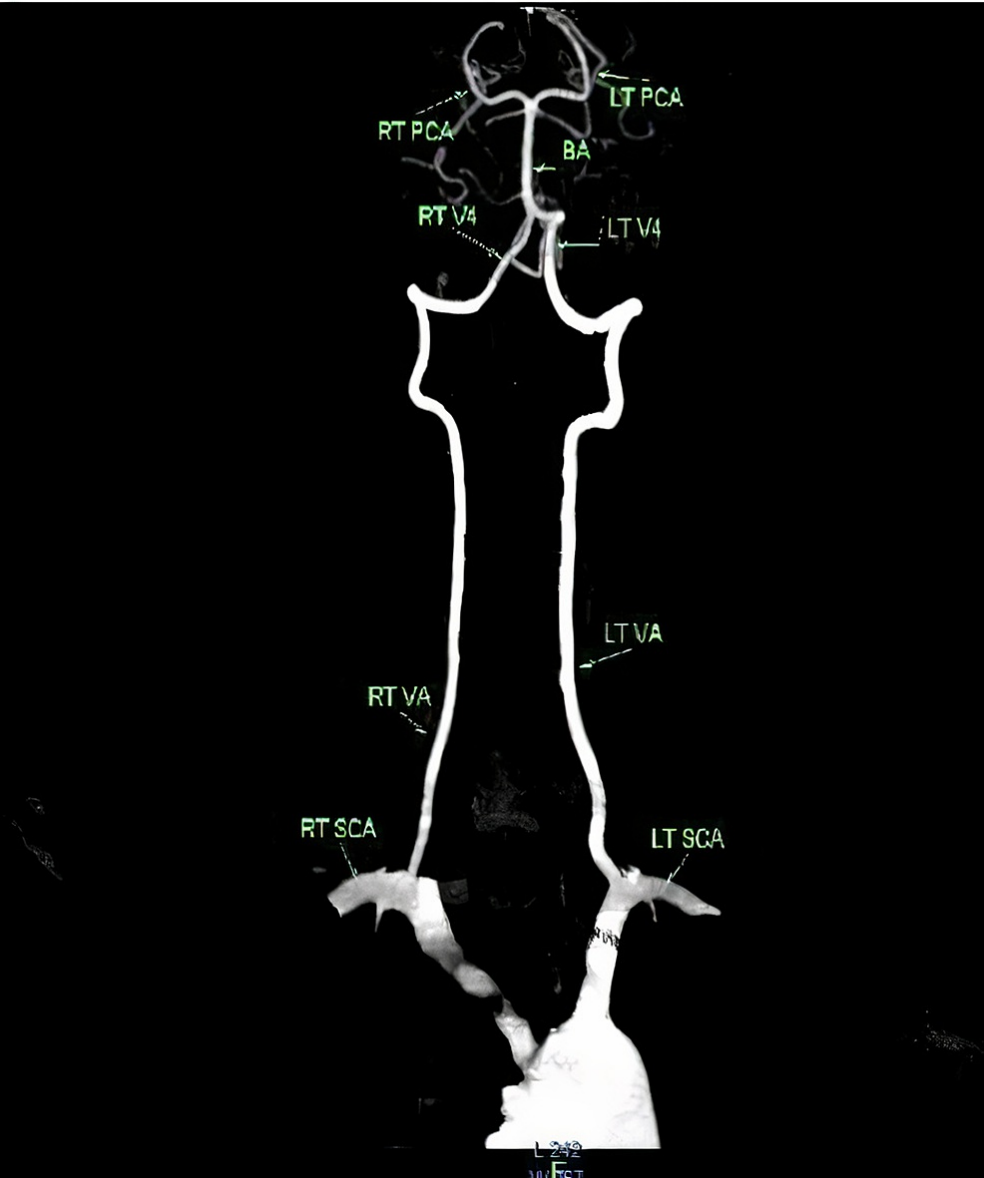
CT image showing the scheme of the vertebral artery as a whole CT - computed tomography
